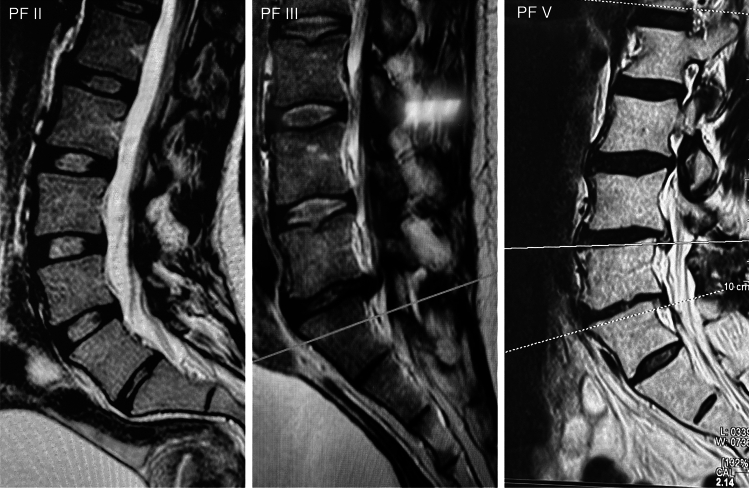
Representative sagittal T2-weighted MR images of IVDs from patients with different Pfirrmann (PF) grades
